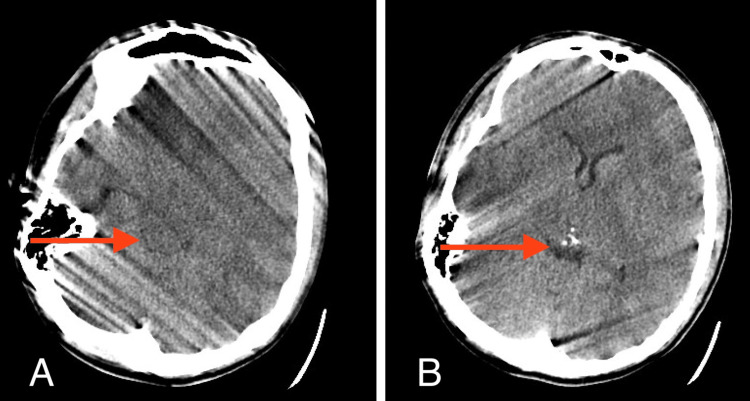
Axial head CT (A, B), obtained immediately after orthopedic fixation showing no abnormalities and open cisterns (red arrows)
